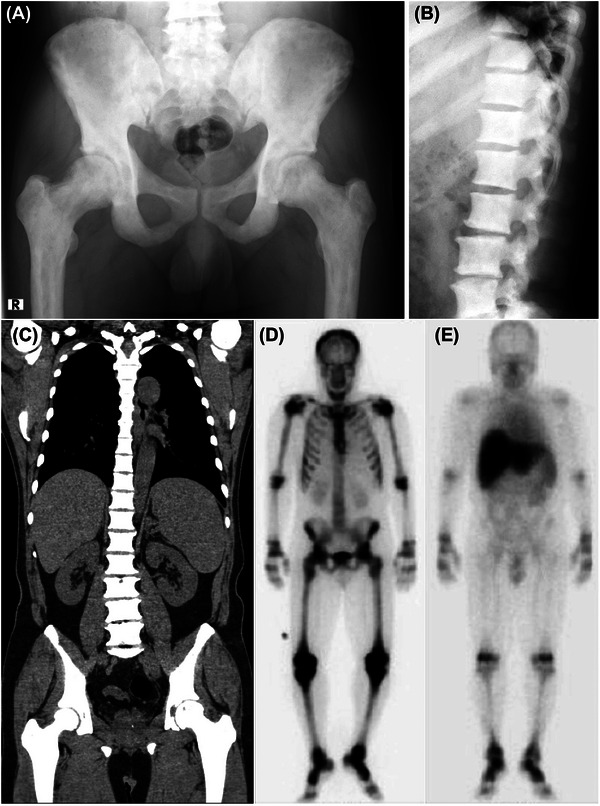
Radiological findings. Plain radiography showed marked calcification of the pelvis (A) and lumbar vertebrae (B). Computed tomography of the body revealed severe systemic osteosclerosis and moderate hepatosplenomegaly (C). Bone scintigraphy showed diffuse high uptakes in systemic bones and joints (D). Bone marrow scintigraphy demonstrated marked accumulation in the liver and spleen, suggesting extramedullary hematopoiesis (E).
